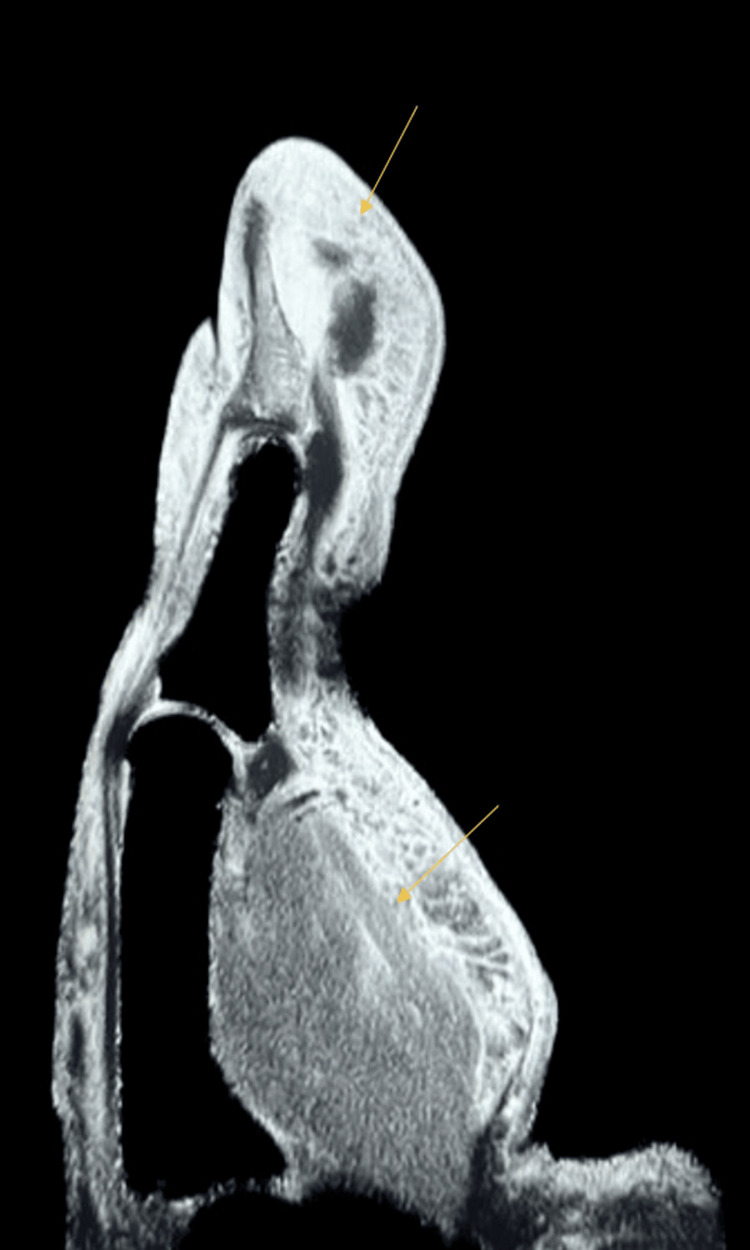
MRI of the first distal phalanx showing soft tissue swelling and edema.
